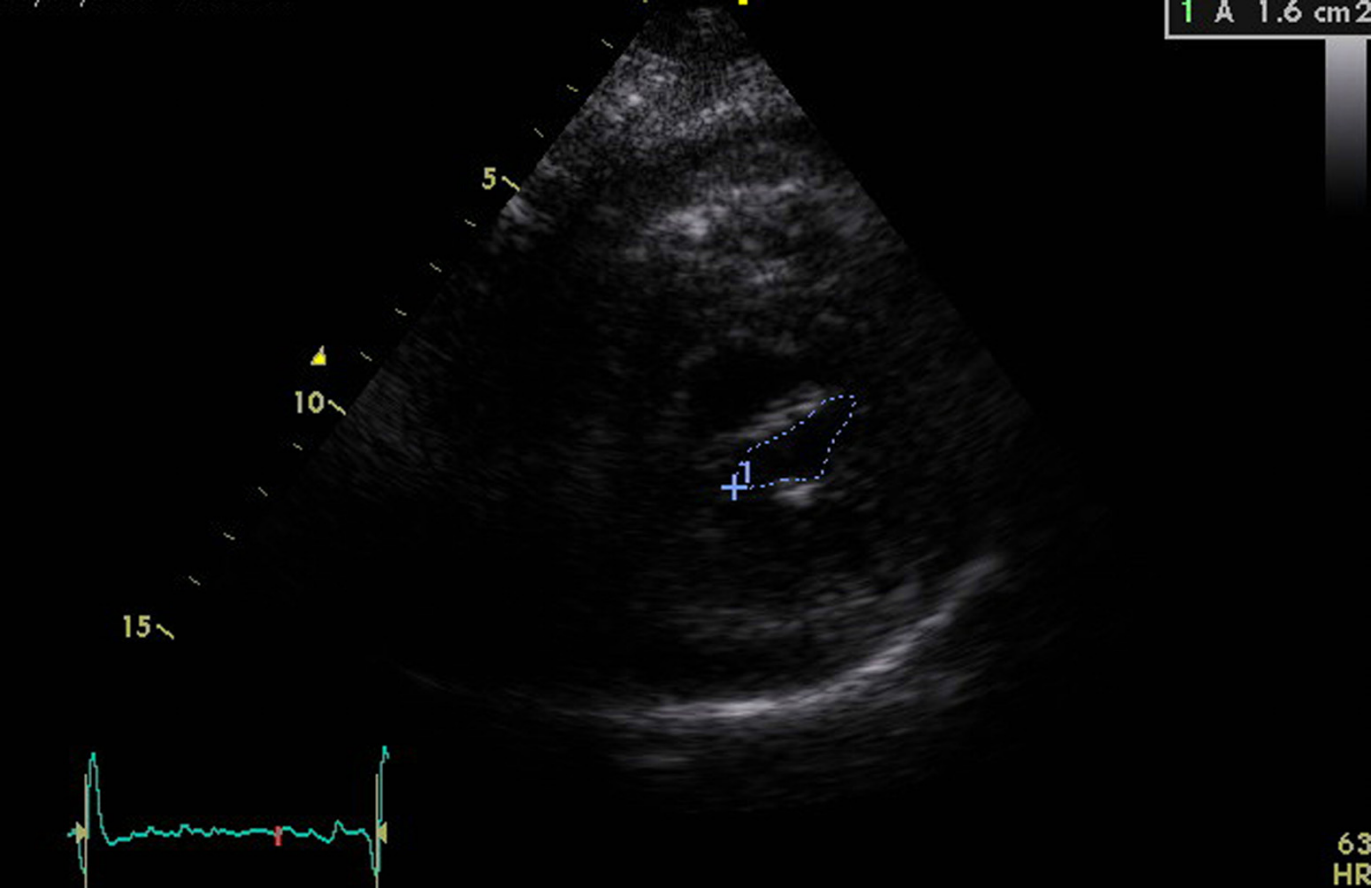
Calculation of mitral valve area(MVA) via planimetry method from parasternal short-axis view in one patient with mitral annulus calcification(MAC) after PTMC.
